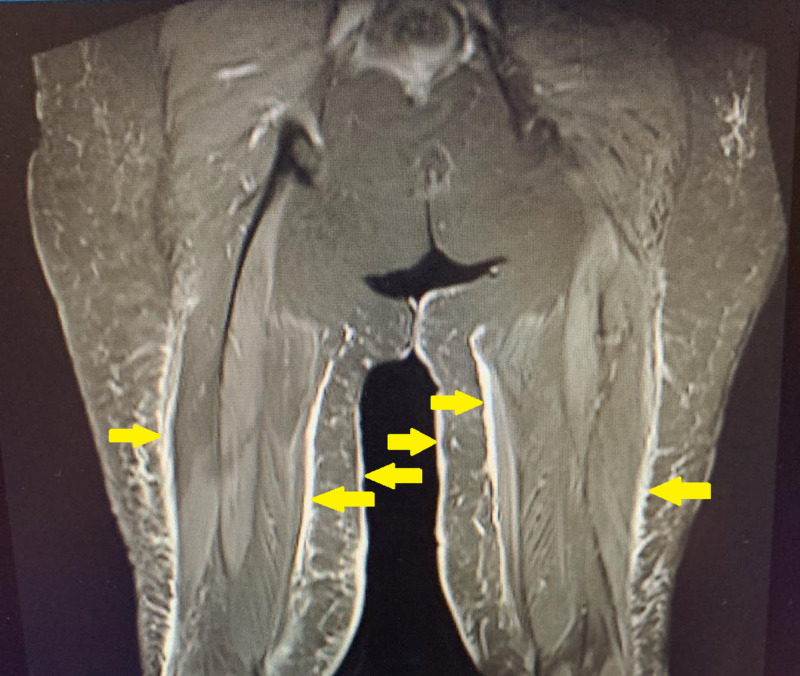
MRI of the hip and thigh showing multi focal myositis of the adductor longus.
